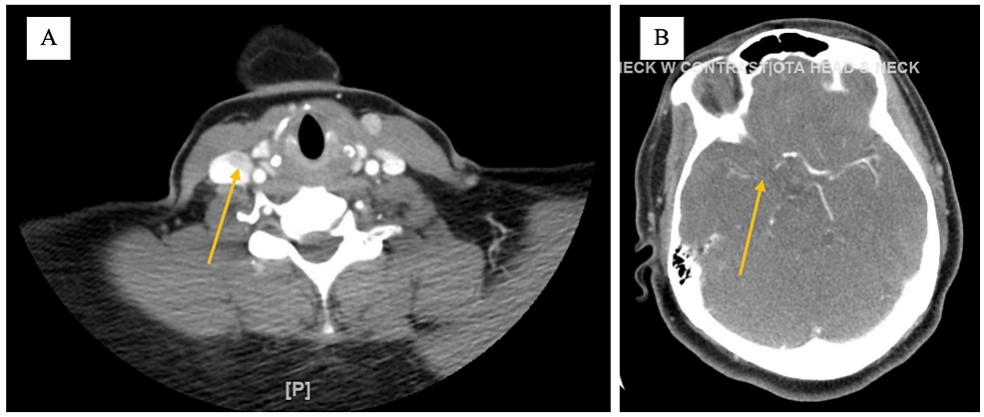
CT angiogram CT angiogram showing (A) occlusion of the supraclinoid internal carotid artery on the right (yellow arrow) and (B) cross-filling of the right A2 segment and the distal M1 segment of the middle cerebral artery (MCA) (yellow arrow).
